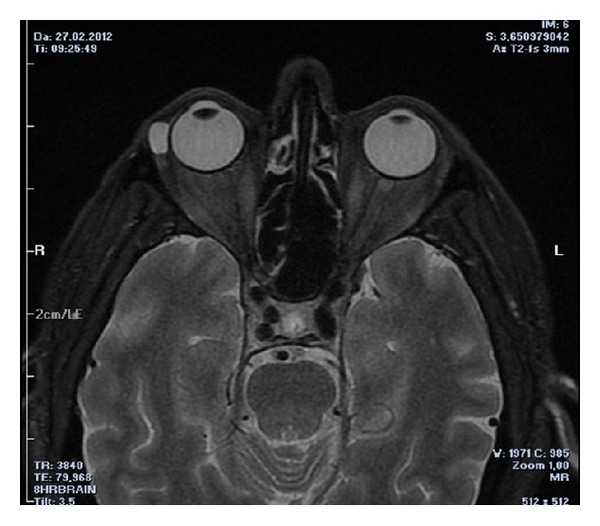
Magnetic resonance image of dacryops.
